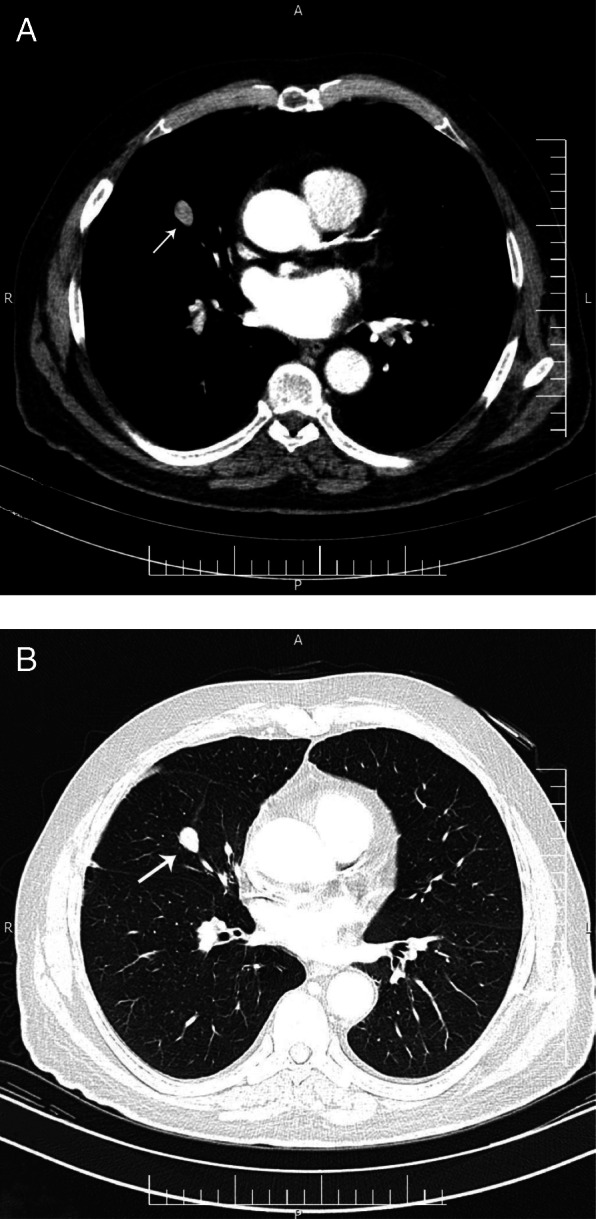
Spiral chest computed tomography of the patient in 2017. a mediastinal view, b parenchymal view of lung nodule (arrow)
